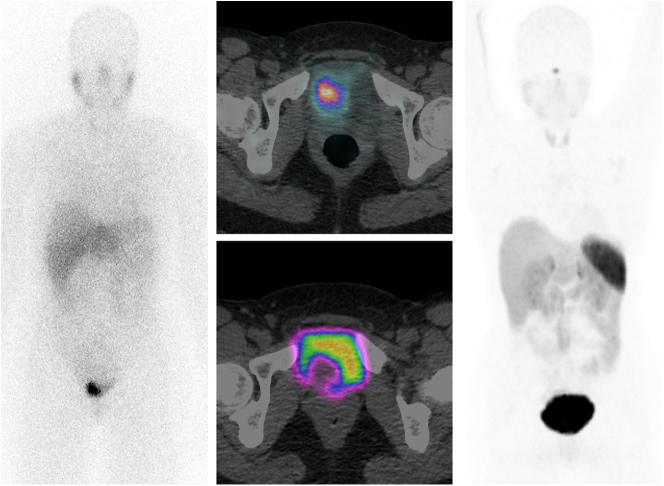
^123^I MIBG whole-body planar scintigraphy and fused axial image at the level of the bladder mass (left and top middle); ^68^Ga DOTATATE whole-body PET/CT three-dimensional maximum intensity projection image and fused axial image at the level of the bladder mass (right and bottom middle). These show the bladder tumor to be showing intense uptake of ^123^I MIBG but little uptake of ^68^Ga DOTATATE. Abbreviations: ^123^I MIBG, iodine-123-metaiodobenzylguanidine; ^68^Ga DOTATATE PET/CT, tetraazacyclododecane tetraacetic acid–DPhe1-Tyr3-octreotate positron emitting tomography.
